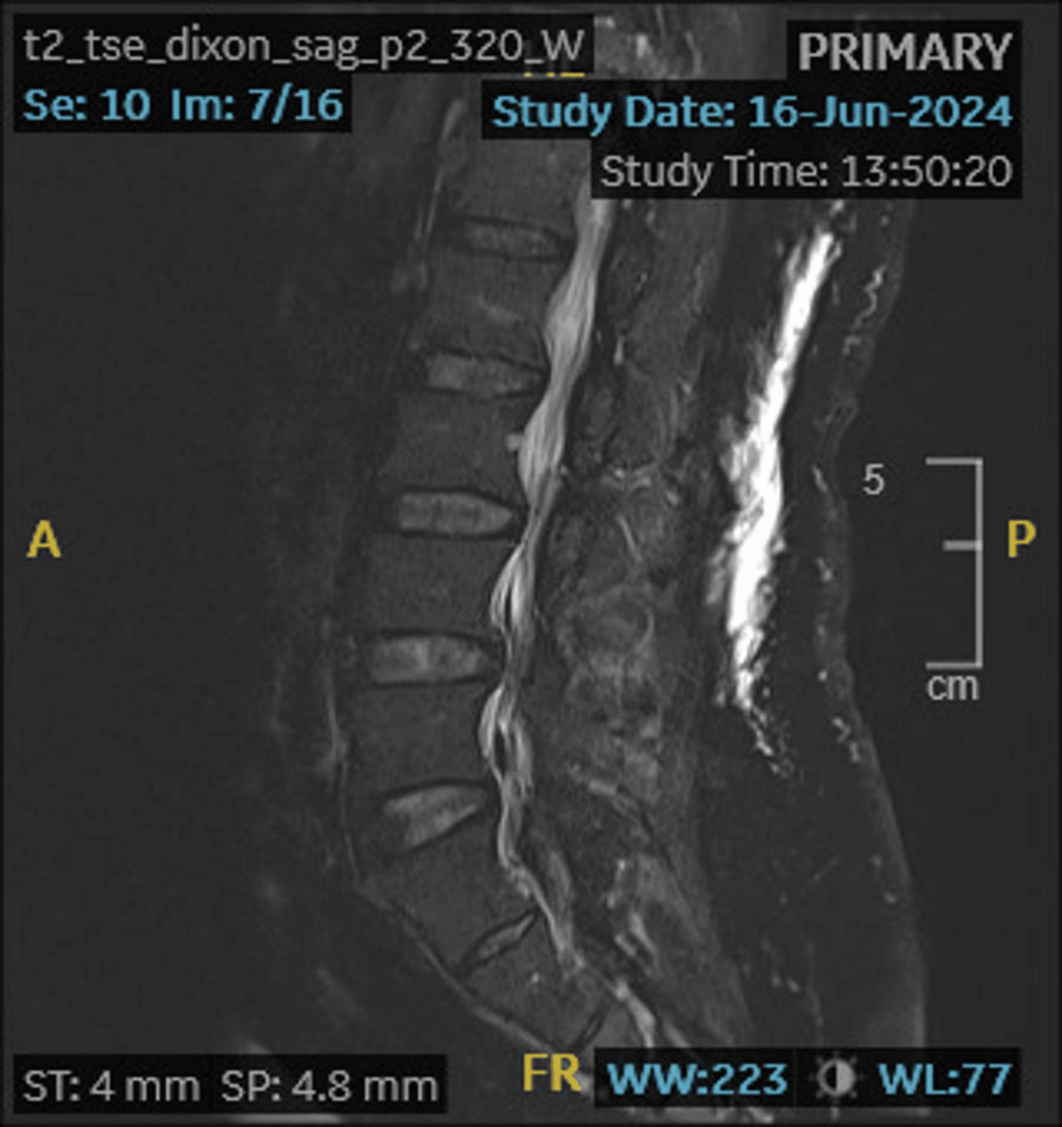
MRI Spine showing disc lesions L3/L4, L4/5 with neural compression
